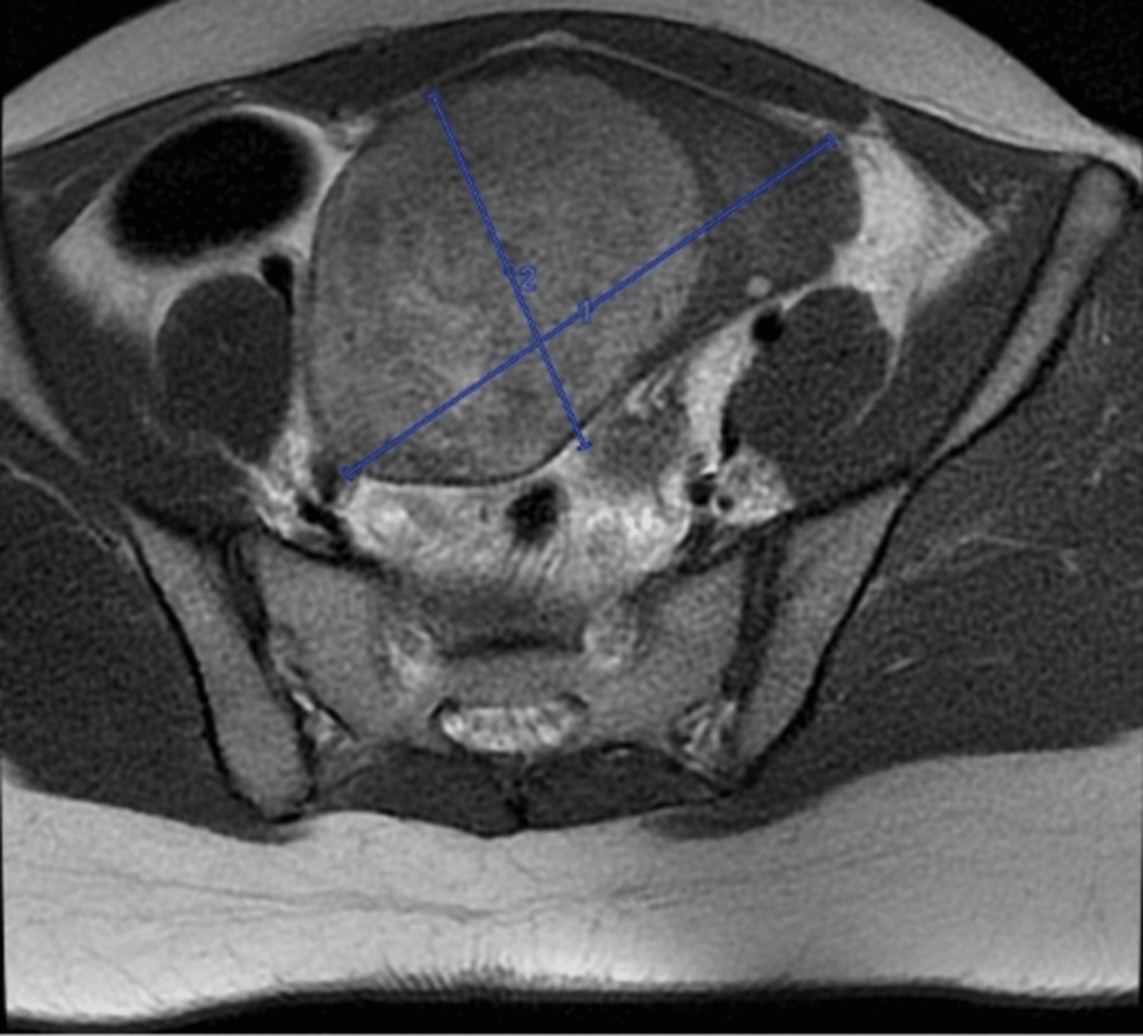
Pelvic MRI (coronal view) highlighting the extent of the solid mass across the right adnexal region into the left adnexal region, indicating its large size and complex nature with tiny cystic components
